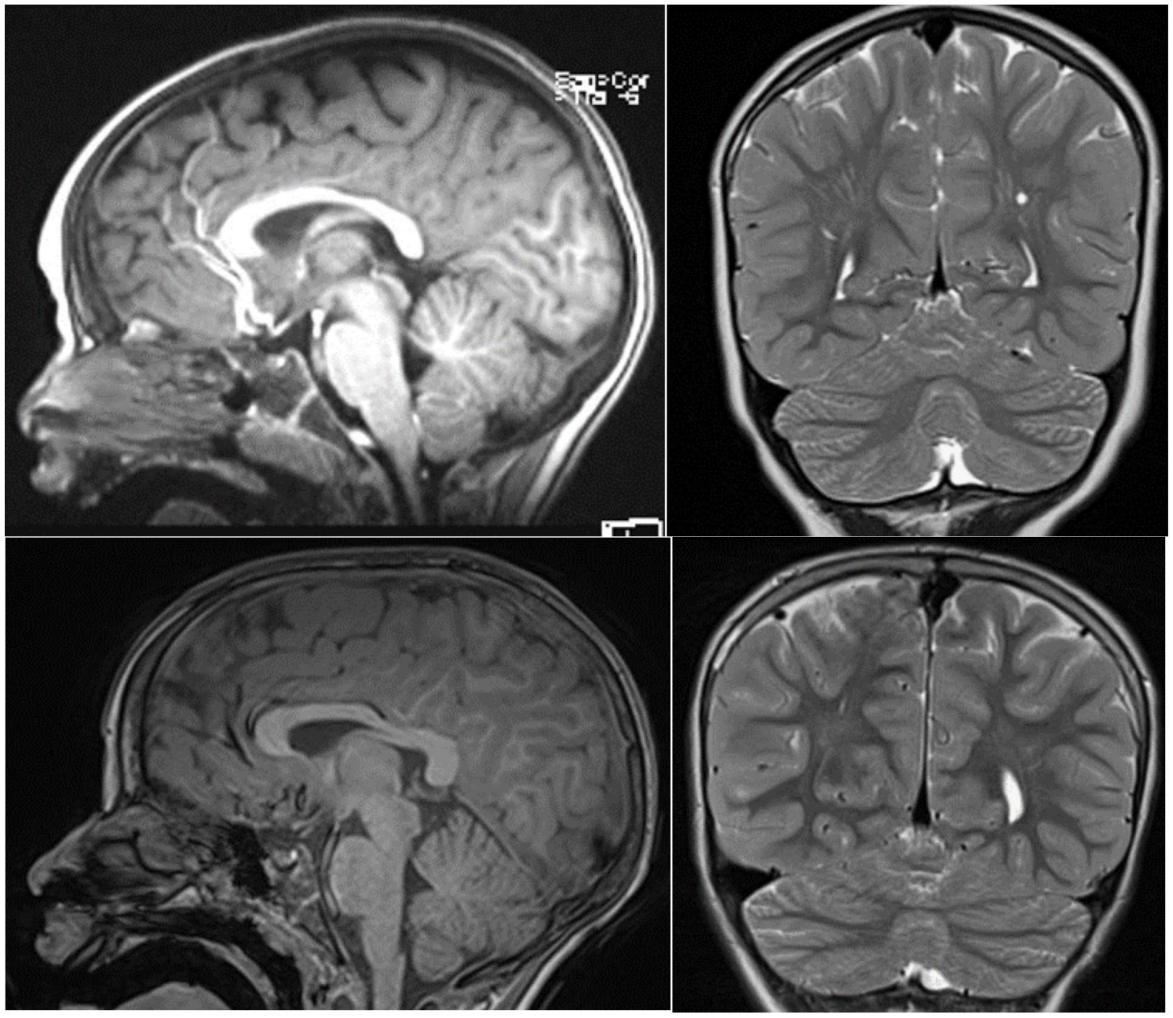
T2-midsagittal and coronal MRI neuroimages showing vermis cerebellar atrophy of patient II-1 F1 upper panel and patient III 1 F2 lower panel.
